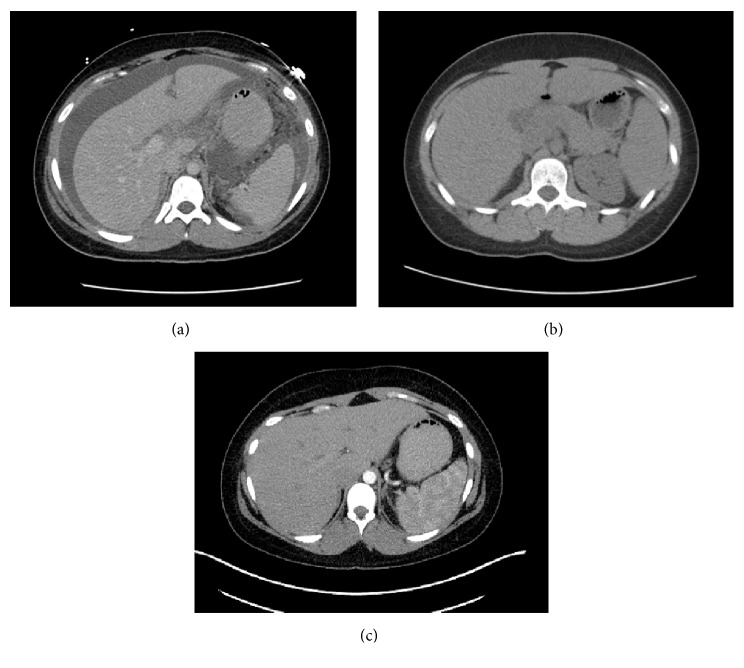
Contrast enhanced CT, axial view, upper abdomen. (a) Completed October 2016 with benign findings. (b) Completed November 2016 with multiple bilobar low attenuation indeterminate hepatic lesions. (c) Completed June 2017 with benign findings.
